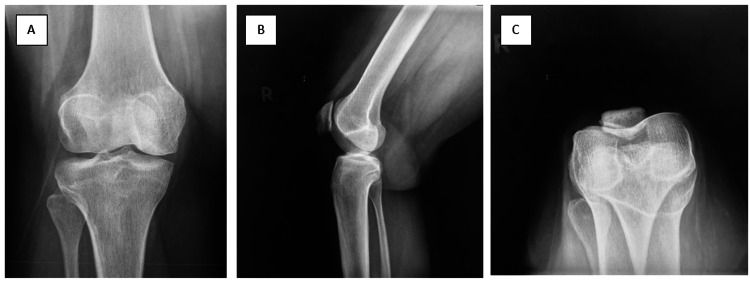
Images A to C showing radiographs of the patient's right knee in AP, lateral, and Merchant's views. AP: Anteroposterior
